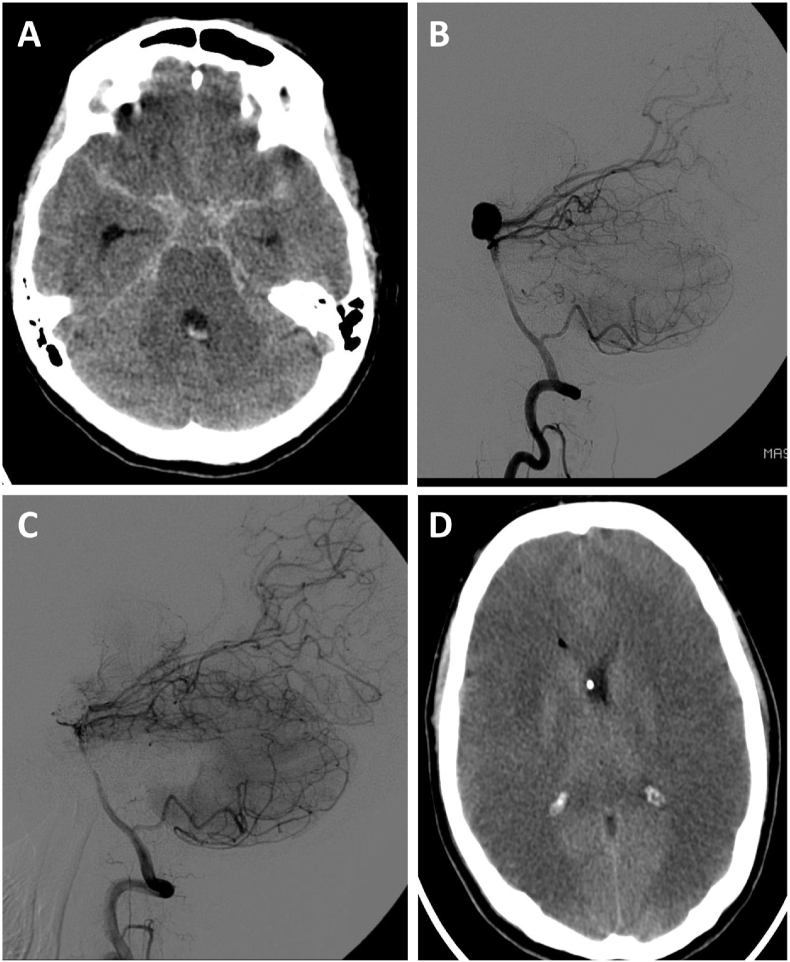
Imaging data from the patient described in our case report of aSAH during pregnancy. (A) Initial CT scan showing symmetric subarachnoid hemorrhage around the circle of Willis and the basal cistern as well as associated hydrocephalus. (B & C) Digital subtraction angiography (DSA) showing a 11 × 9 mm saccular basilar tip aneurysm before (B) and after (C) coil embolization of the aneurysm. (D) CT scan showing bi-hemispheric infarction of the middle cerebral artery (MCA) and anterior cerebral artery (ACA) areas as well as an associated bi-hemispheric cerebral edema with compression of the ventricular system.
